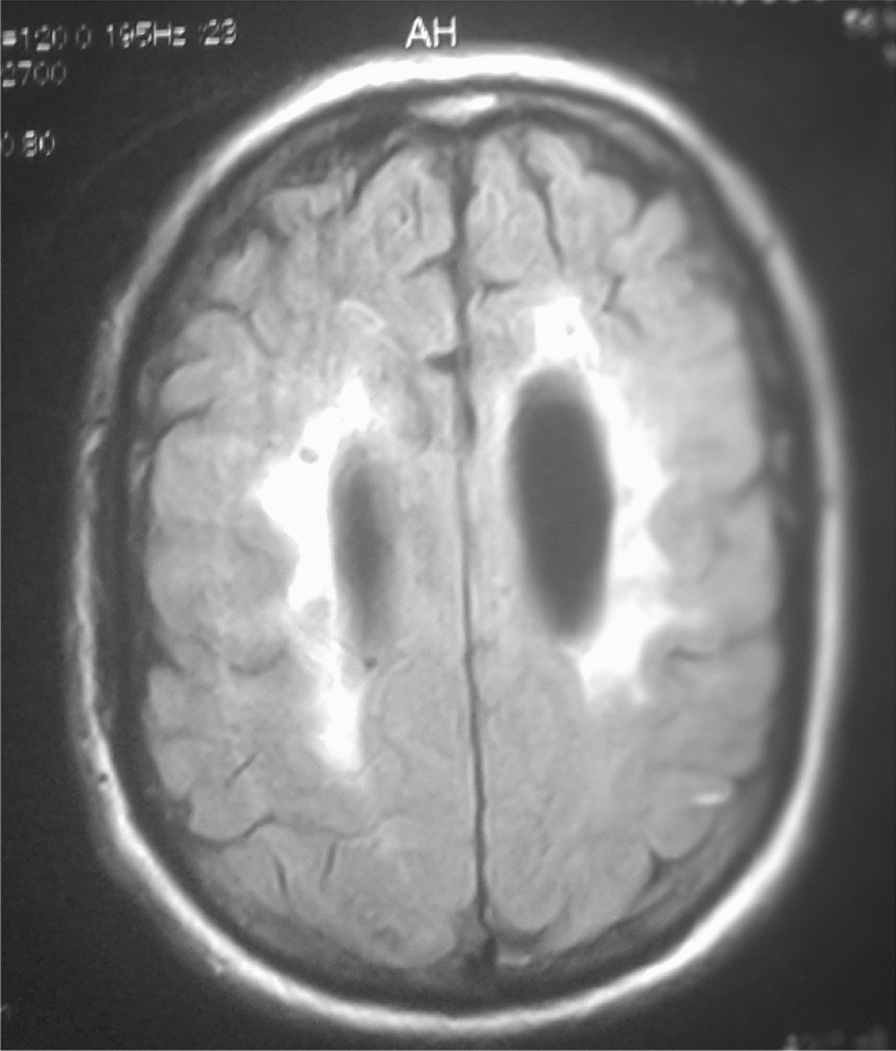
Cerebral MRI: focal abnormality in subcortical white matter, periventricular hyperintensities on T1-weighted image
